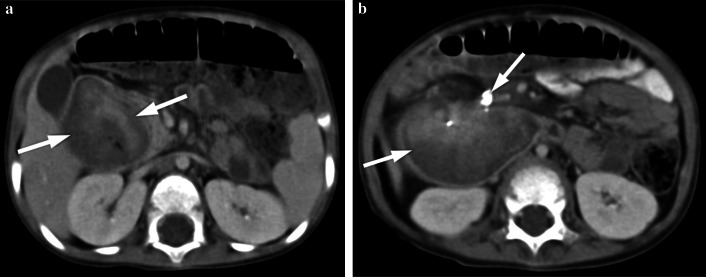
a, b A 3-year-old boy with duodenal gastrointestinal stromal tumor who had been complaining of vomiting for 3 months. The mass was palpable in physical examination. a, b Contrast-enhanced axial CT images show duodenal tumor with calcifications (arrows)
